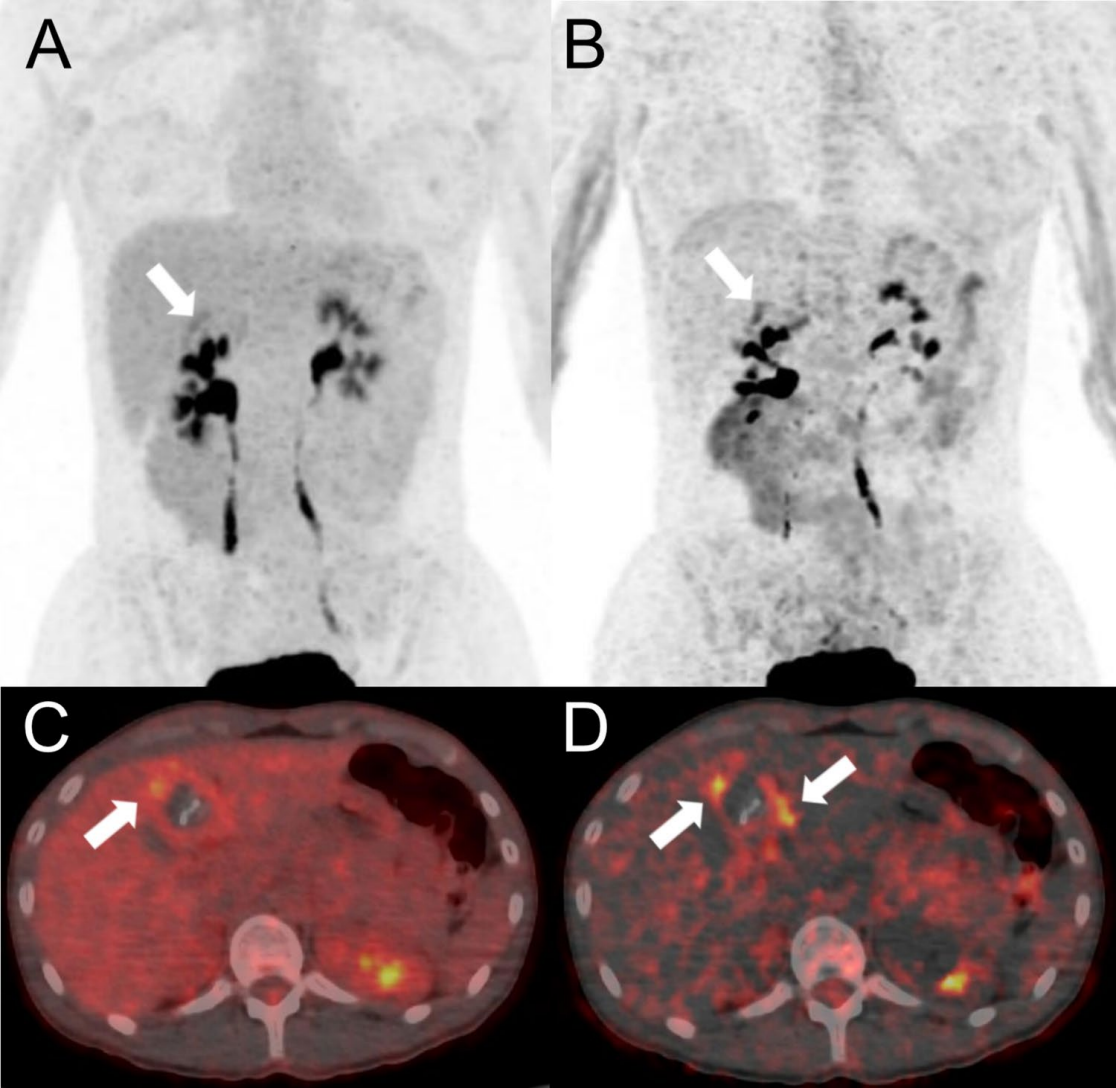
PET/CT performed for staging of AE in a 26-year old woman (patient 10 in Table 1) showed mild metabolic activity (in maximum intensity reconstructions of PET, A and B) and fused PET/CT images (C and D) in a partially calcified lesion in the left liver lobe. In standard static PET/CT images (A and C), SUVmax of the AE manifestation was 4.1, and the lesion-to-background ratio (SUVratio) was 1.3. For WBD PET/CT (B and D), the respective value in the AE manifestation was 15.7, and the lesion-to-background ratio was comparatively higher (Patlak ratio 2.6)
